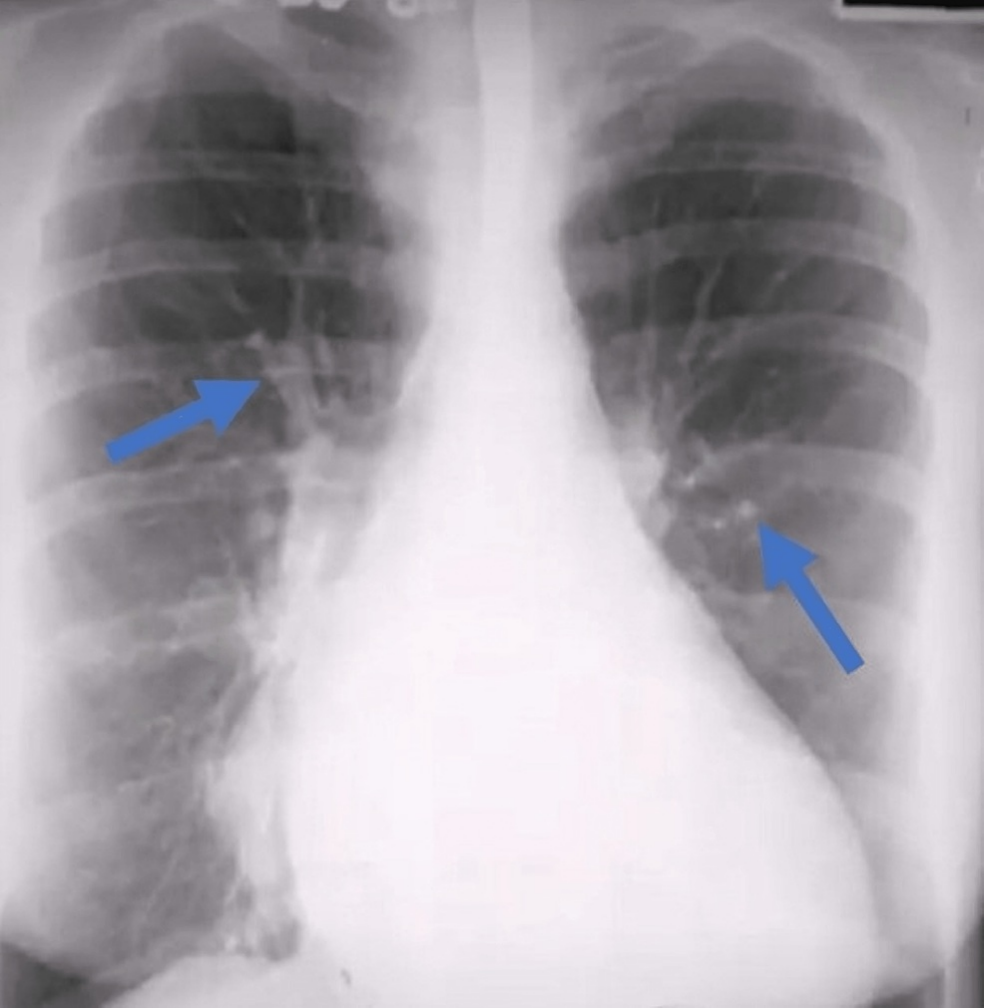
Chest X-ray revealing cardiomegaly, vascular redistribution, and interstitial edema.
